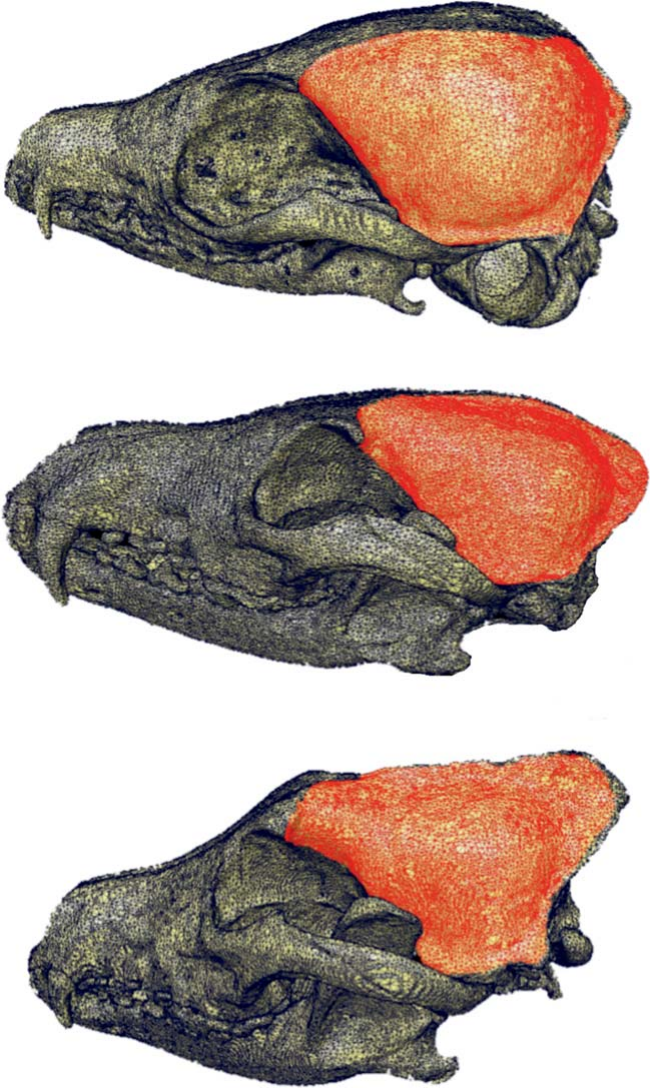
3D CT reconstructions demonstrating the area of origin of temporalis (red) on three species. A, *Vulpes zerda* exhibits a wide sagittal gap where left and right temporalis do not meet at midline, B, *Vulpes vulpes* demonstrates that the temporalis origin utilises all of the dorsal calvarium, and C, *Canis lupus*, displays a pronounced sagittal crest for increasing the surface area of temporal attachment.
